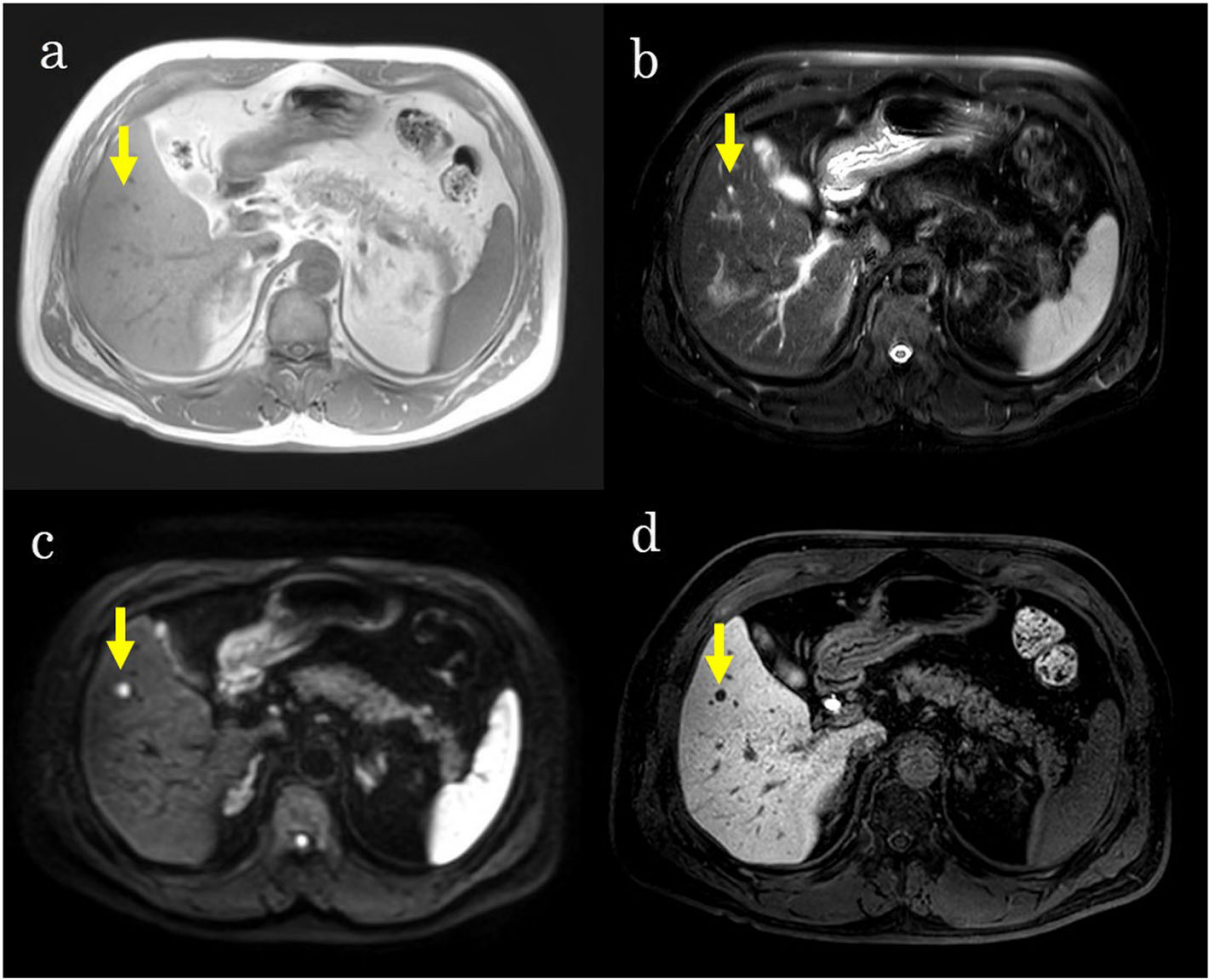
Magnetic resonance imaging (MRI) 1 year after abdominoperineal resection. a The tumor in segment 6 of the liver (arrow) showed low intensity on T1-weighted images. b The tumor in segment 6 of the liver (arrow) showed high intensity on T2-weighted images. c The tumor in segment 6 of the liver (arrow) showed high intensity on diffusion-weighted images. d The tumor in segment 6 of the liver (arrow) showed low signal intensity on gadolinium-ethoxybenzyl-diethylenetriamine pentaacetic acid-enhanced MRI (EOB-MRI)
